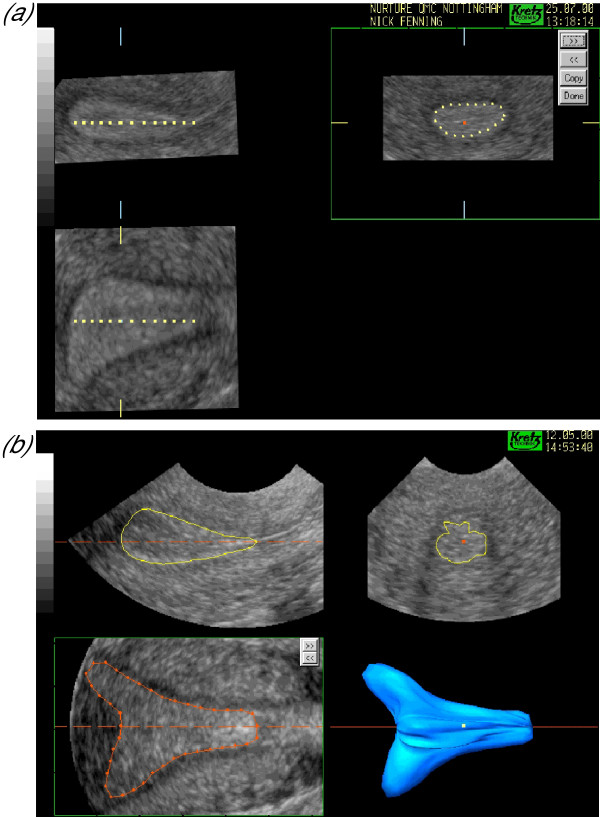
The techniques of volume calculation*. Both figures show the typical multiplanar display of a three-dimensional sonographic dataset of the uterus with the three mutually related orthogonal planes at 90-degrees to one another. The upper left image in both displays represents the longitudinal plane (the A plane), the upper right image the transverse plane (the B plane) and the lower left image the coronal plane (the C plane). Volume calculation can be conducted in either the B or C plane. (a) shows the conventional technique of volume calculation in which a series of 'slices' are taken through the volume of interest whilst the contour is outlined in another plane (the transverse plane in this case). The distance between consecutive slices can be varied according to the degree of change in the surface contour and increased for more complex structures. (b) shows the rotational technique of volume calculation in which the dataset is rotated through 180° about a central axis defined by the application of two callipers. The number of planes available for volume calculation are determined by the rotation step shown in the lower left of the image. Here the 30-degree rotation step has been used and the contour outlined in the coronal or C plane using the manual mode. The resultant three-dimensional model is shown in the lower right of the image.
